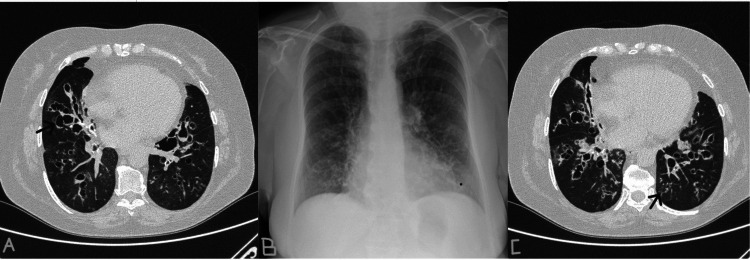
Chest CT images obtained in April 2025 (A) Axial view showing bilateral varicose-saccular bronchiectasis predominantly in the lower lobes; arrows indicate representative areas of bronchiectasis. (B) Coronal reconstruction highlighting cystic changes and bronchial wall thickening. (C) Sagittal section demonstrating associated reticulo-micronodular infiltrates suggesting chronic infectious-inflammatory changes.
